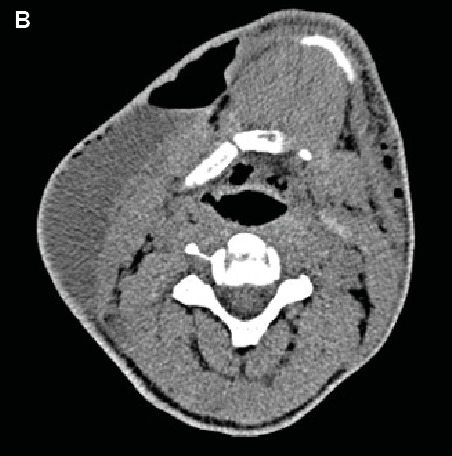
A CT scan shows a large lesion of fluid density on the right side of the neck.
